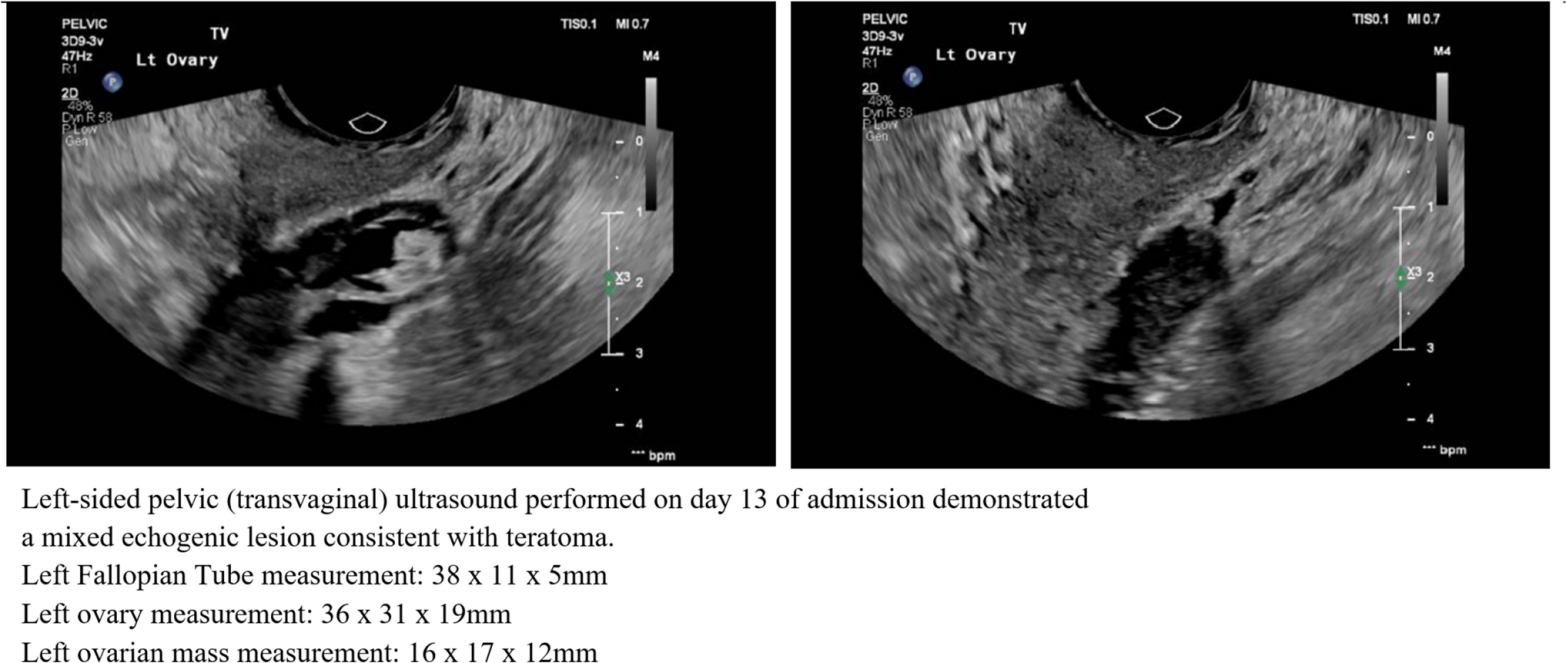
Left-sided pelvic ultrasound
